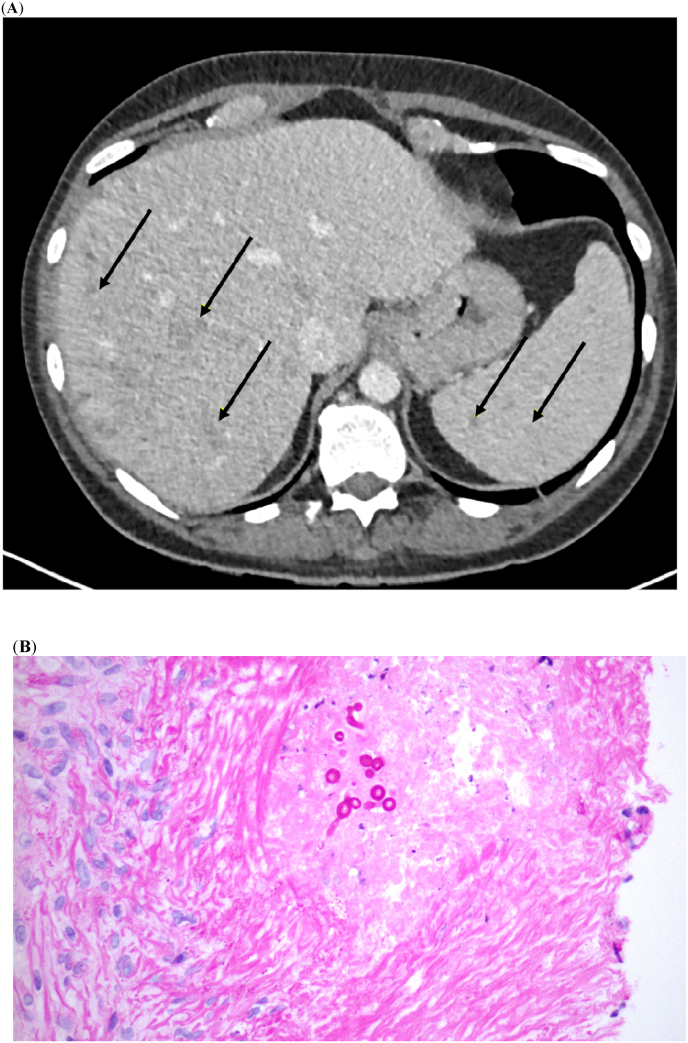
Case two: (A) axial image from a portal phase CT demonstrating (arrows) numerous hypo-attenuating lesions in the liver and spleen, (B) liver biopsy with parenchyma showing a single large focus of coagulative necrosis with palisaded epithelioid histiocytes, fibrosis, lymphoplasmacytic infiltrate and a few fungal cells with short hyphae.
